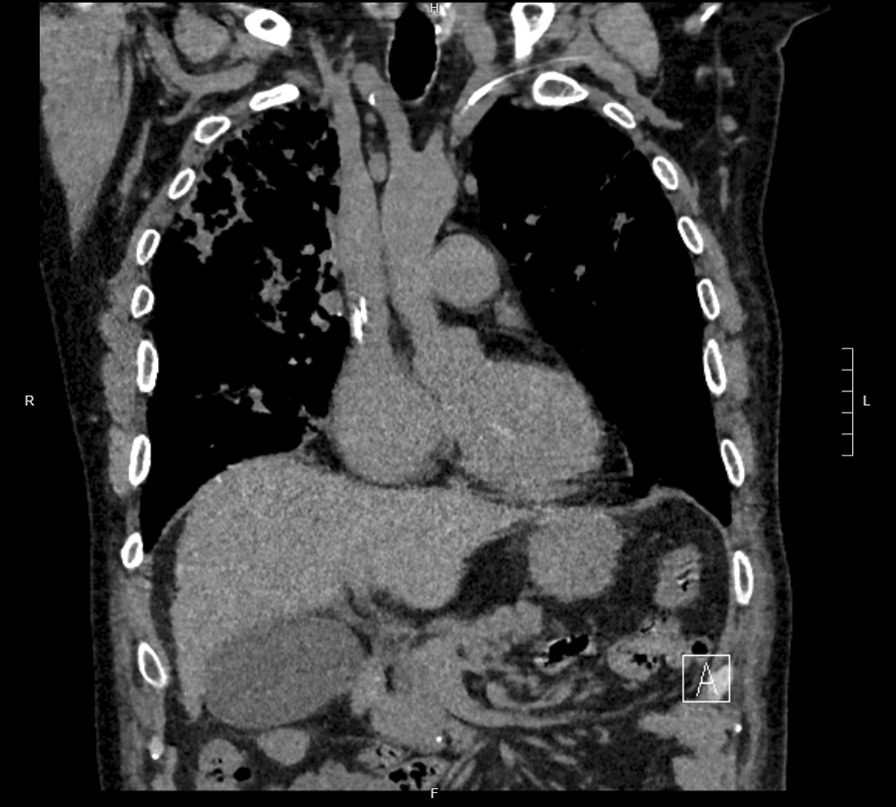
CT high resolution—chest
